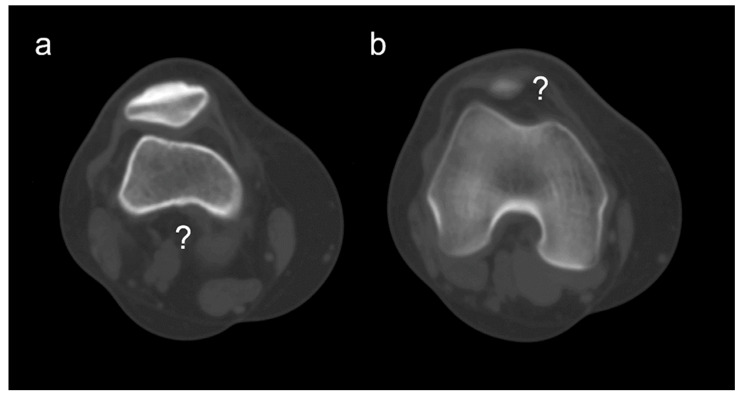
Axial CT images of the patellofemoral joint in two patients with patella alta. In the first patient, the posterior intercondylar notch cannot be visualized on the same axial slice as the patella (a), whereas in the second patient, the patella cannot be visualized on the same slice as the posterior intercondylar notch of the femur (b).
